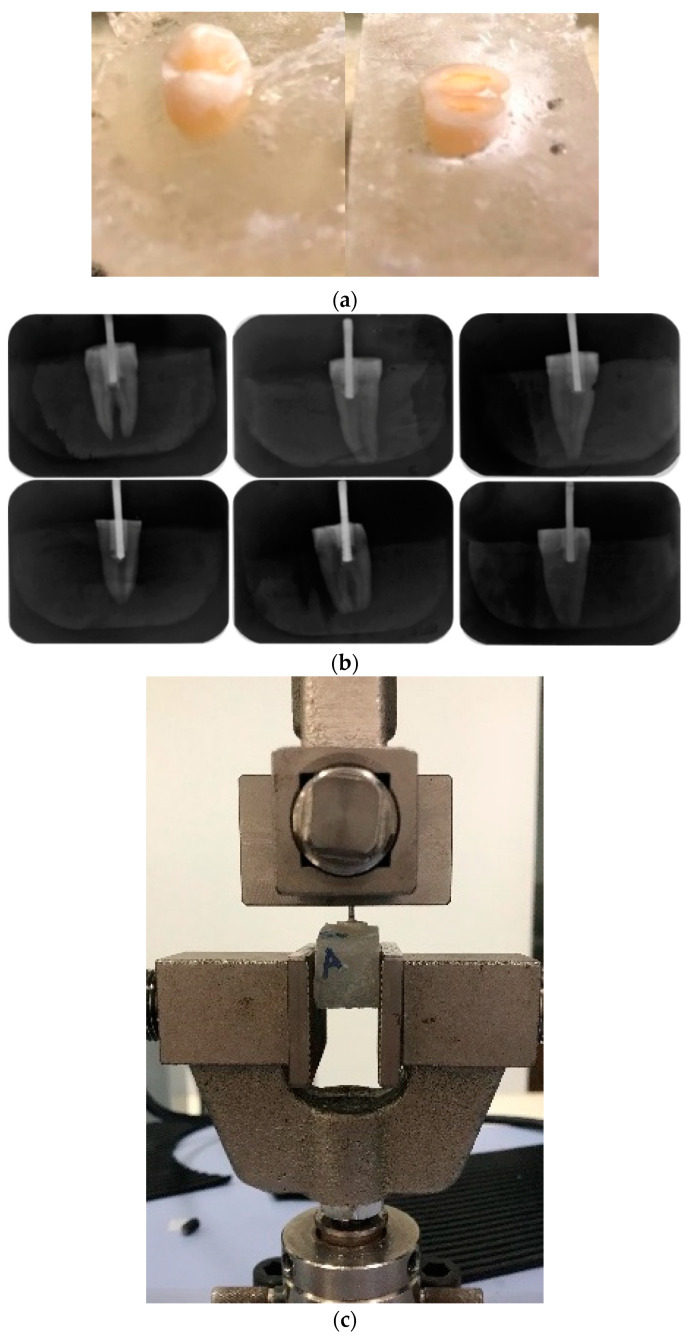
Pull-out test for measuring adhesion strength. (a) Premolars embedded in an acrylic resin. (b) Radiographs of premolar with metal post. (c) Pull-out test.
